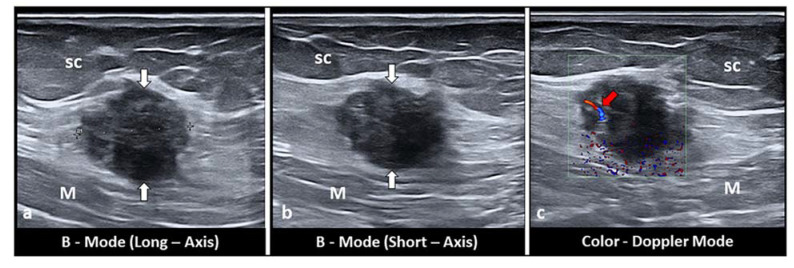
Long-axis (a) and short-axis (b) sonograms show a hypoechoic, nodular mass (white arrows), located between the subcutaneous tissue (sc) and the muscular plane (M) of the abdominal wall. Of note, fine intralesional, vascular spots (red arrow) are depicted on the color Doppler (c).
